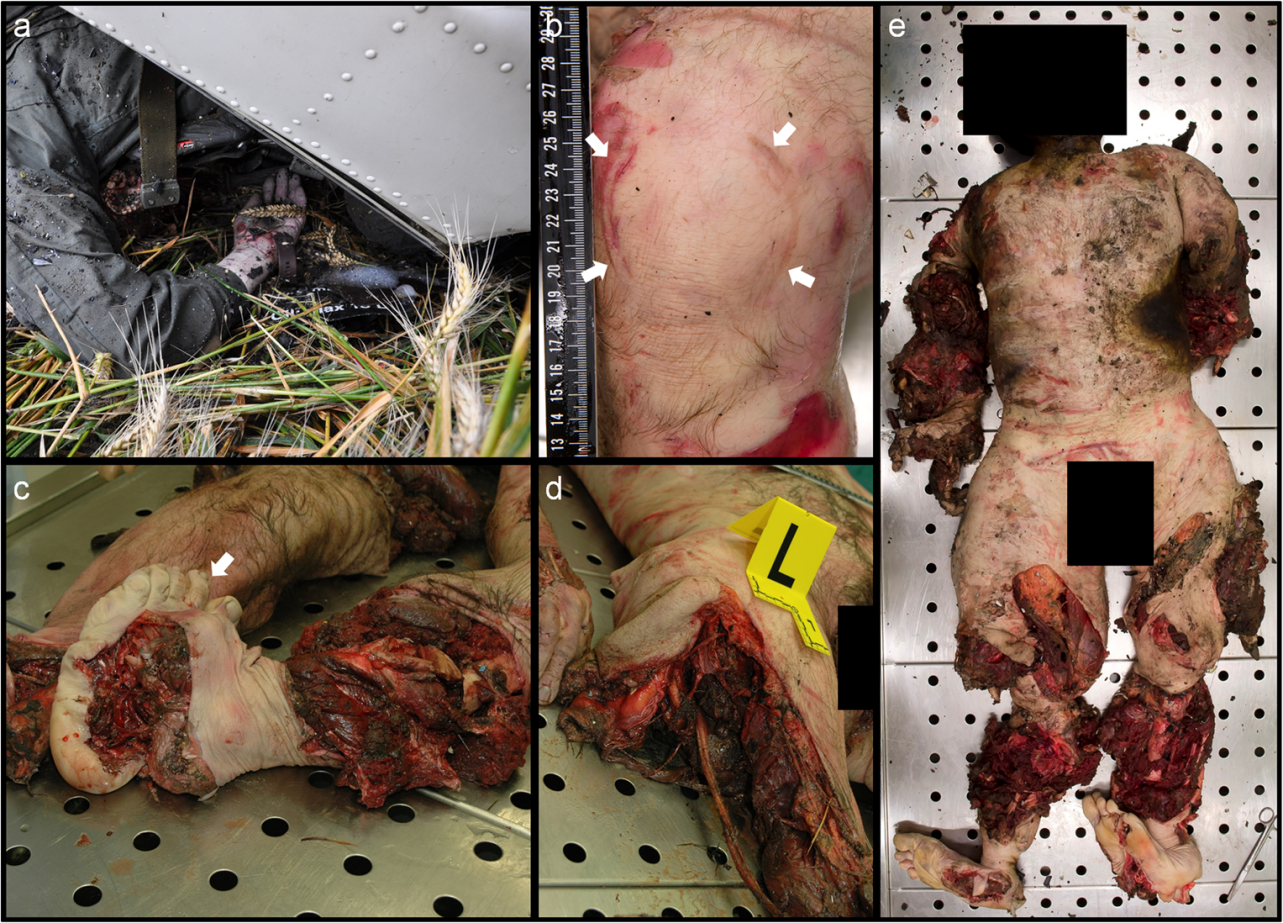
Images of various victims from small aircraft crashes a Picture of a detail of the victim, taken at the scene of the crash. The hand and part of the aircraft are recognizable. b Ventral view of a bended knee. Note the round traumatic impression (arrows), most likely caused by hitting the dashboard. c Extensive destruction of the left lower leg and right upper extremity and both feet. The toes of the left foot are recognizable (arrow). d A large laceration of the right upper extremity. e Ventral total body view. Note the destruction of all four extremities in contrast to the seemingly intact pelvic region. Typical telescopic shortening of the legs [15]. A 3D–CT scan of this victim is shown in Fig. 3h
